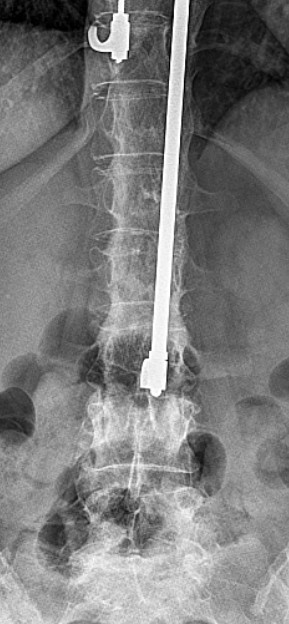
X-ray of the lumbar spine in anterior-posterior projection
